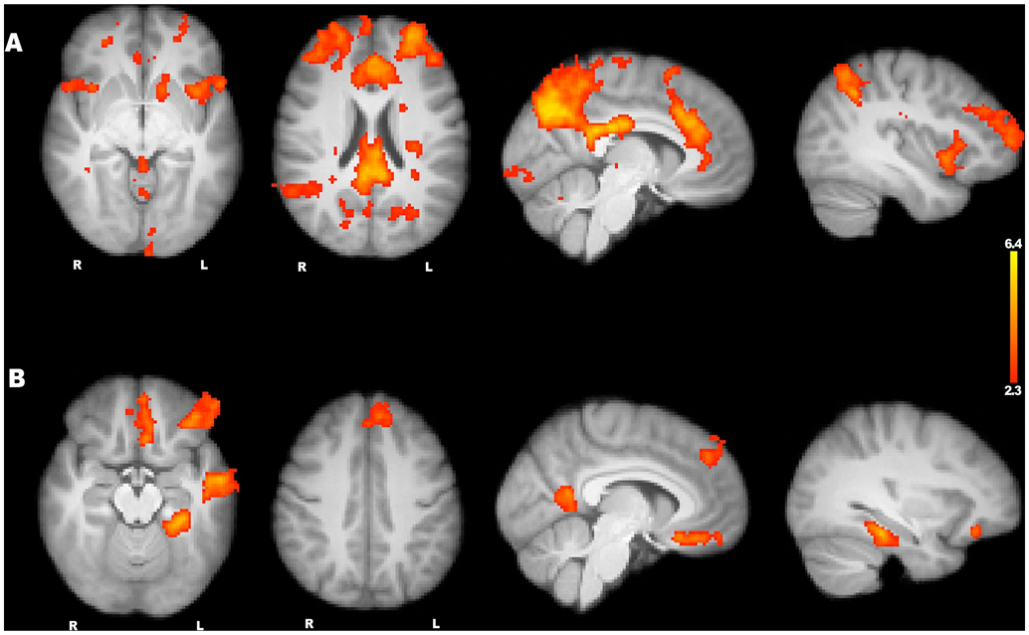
Religious versus nonreligious statements. (A) The MRI signal was greater when subjects evaluated religious statements compared with nonreligious statements in areas throughout the brain, including the precuneus, anterior cingulate, insula, and ventral striatum. (B) Increased signal was found for nonreligious statements compared with religious statements in several left hemisphere regions including the parahippocampal gyrus, retrosplenial cortex, temporal pole, middle temporal gyrus and hippocampus.
